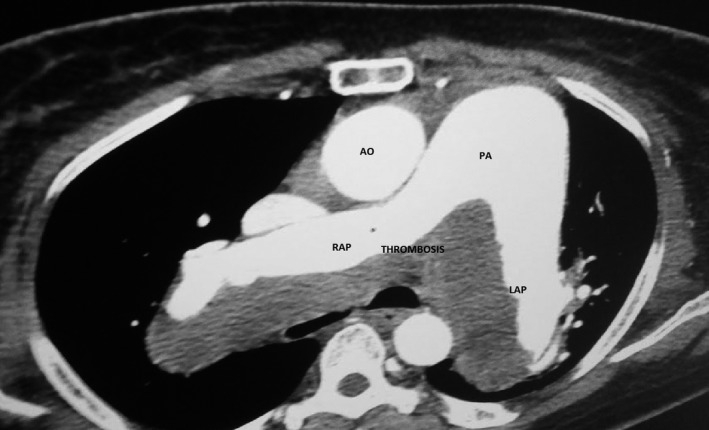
Chest CT scan showing the dilatation of the pulmonary artery trunk (diameter measured at 46 mm) of its right (diameter measured at 42 mm) and left (42 mm diameter) branches and intra‐artery thrombosis
